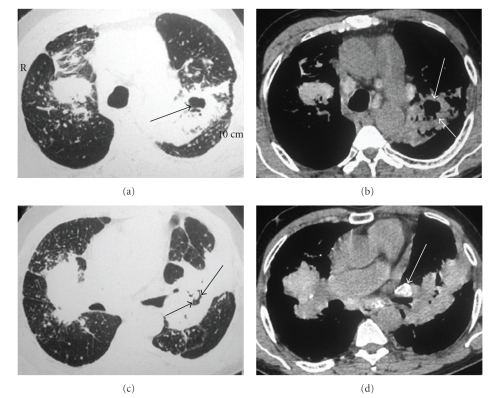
HRCT scan with pulmonary windows ((a) and (c)) showing conglomerate mass (progressive massive fibrosis) with adjacent small nodules in both lungs. Note that the left conglomerate mass presented with a cavitation (arrows). Areas of emphysema and an enlargement of the pulmonary artery trunk are seen. Mediastinal windows ((b) and (d)) also show calcifications in the hilar and mediastinal lymph nodes. Foci of calcification are present in the conglomerate masses.
